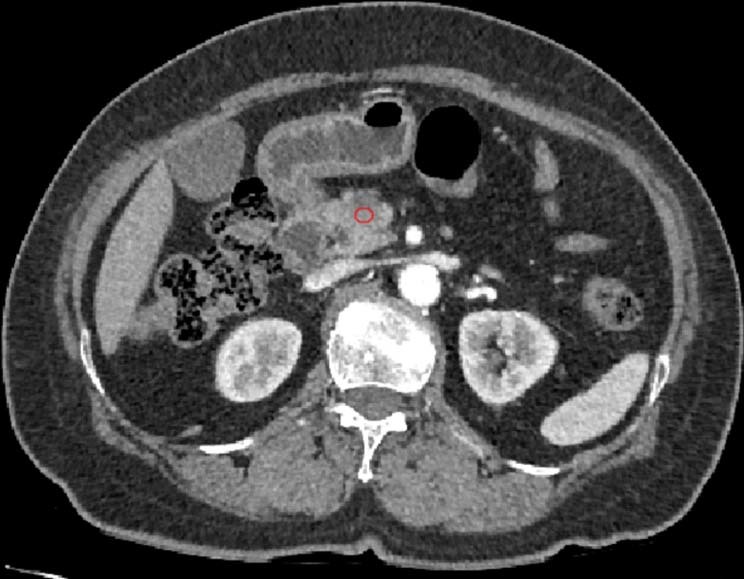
ROI processing in preoperative CT scan.
